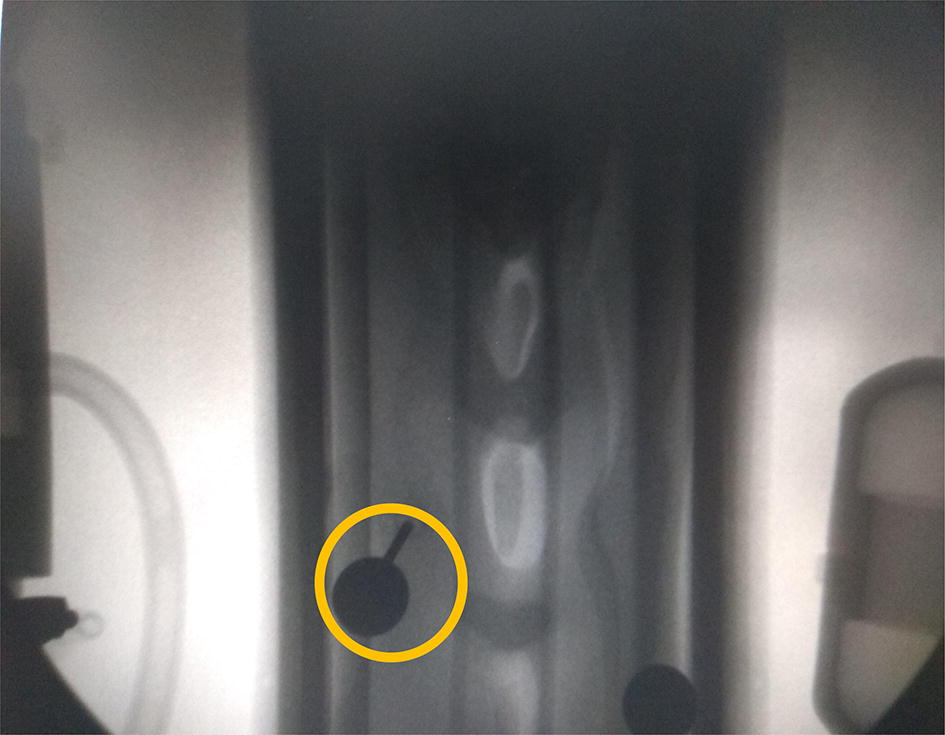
Verification of the effect of the robot puncture mark point by X-ray image
